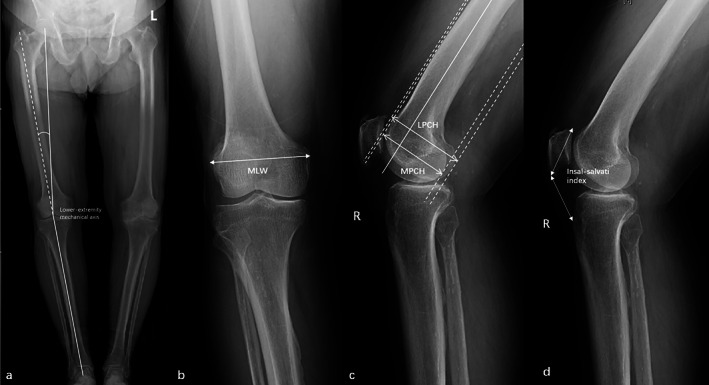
Measurement of the radiograph factors. (A) The mechanical axis was defined as the center of the plateau to the center of the plafond, and the anatomic axis was defined as the center of the tibial diaphysis. (B) MLW: medial‐lateral width of epicondyle. The MLW was defined as the distance between the lateral epicondyle and the medial epicondyles. (C) MPCH: medial posterior condyle height, LPCH: lateral posterior condyle height. The longitudinal posterior condylar line was determined on the cut with the largest anterior–posterior dimension at the lateral and medial femoral condyle. The distance between the anterior femoral cortex line and the posterior condylar line of the lateral and medial femoral condyle was defined as LPCH or MPCH. (D) Insall–Salvati index: The Insall–Salvati index was defined as the Patellar length compared to the patellar tendon length
